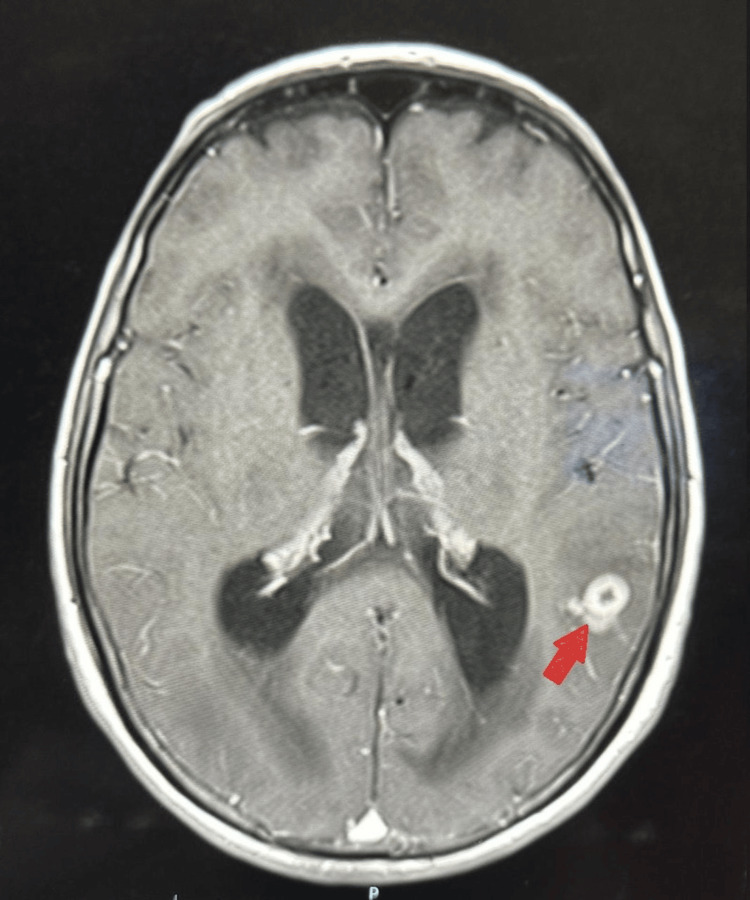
T1-weighted image: communicating hydrocephalus with ventricular dilatation (red arrow)
